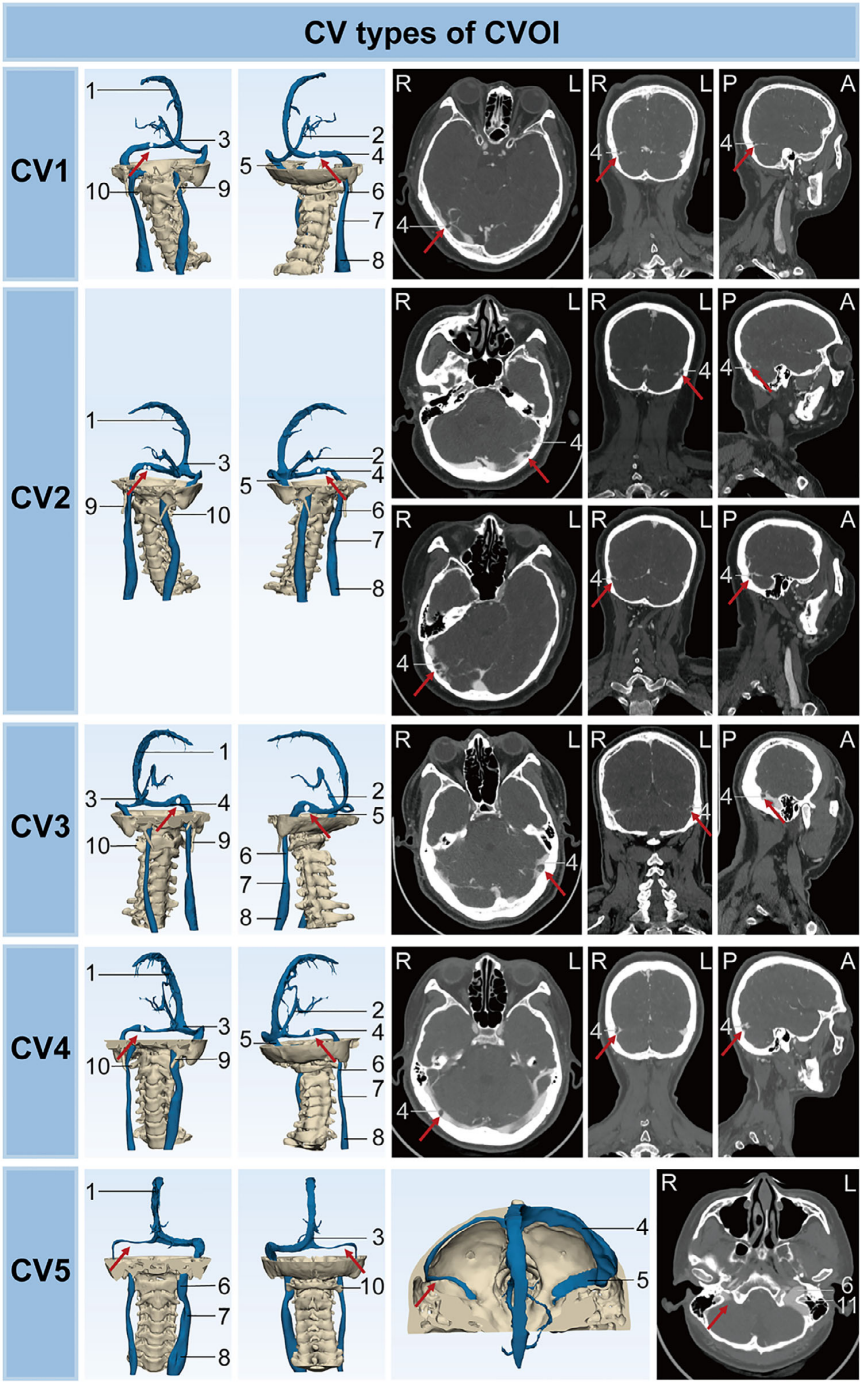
Imaging characteristics of CV types of CVOI on CT. Numbered labels: 1 = superior sagittal sinus, 2 = inferior sagittal sinus, 3 = confluence of sinuses, 4 = transverse sinus, 5 = sigmoid sinus, 6 = internal jugular vein (J3 segment), 7 = internal jugular vein (J2 segment), 8 = internal jugular vein (J1 segment), 9 = styloid process, 10 = transverse process of the atlas (C1), 11 = jugular foramen. CVOI, cerebral venous outflow insufficiency; CV, intracranial type of CVOI; R, right; L, left; P, posterior; A, anterior.
